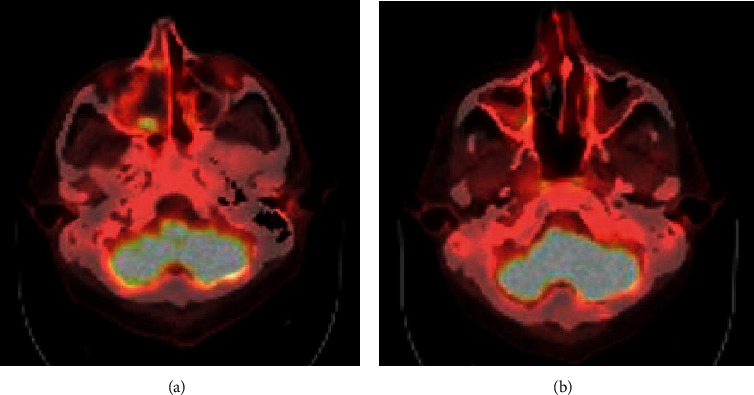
PET/CT scan highlighting the maxillofacial structures. (a) After 2 cycles of mSMILE, a single 1.1 cm focus of increased FDG uptake was seen along the right sinonasal cavity, SUV max 9.3. (b) Restaging PET/CT scan after 6 cycles of mSMILE showing mild residual uptake in the right maxillary sinus, SUV max 4.2.
